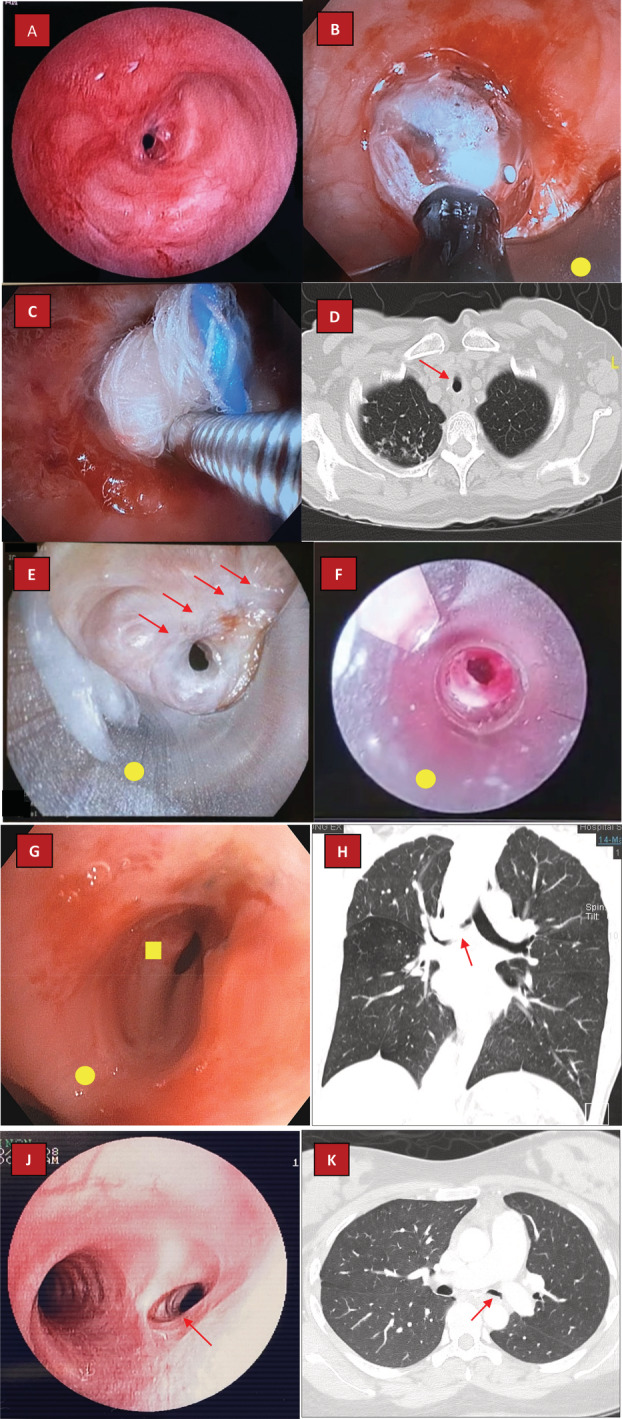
Computed tomography (CT) and bronchoscopy images. (A) Flexible bronchoscopy demonstrating short‐segment concentric membranous tracheal stenosis with opening of approximately 2 mm. (B) Attempting balloon dilatation of stenotic segment under rigid bronchoscopy (bevel of rigid bronchoscope marked with yellow circle). (C) Application of topical mitomycin‐C (TMC) at stenotic segment using soaked gauze balls post balloon dilatation. (D) CT demonstrating stenosis at mid‐trachea (red arrow) due to endobronchial tuberculosis (EBTB). (E) Bronchoscopy demonstrating severe tracheal stenosis with opening of 2 mm. A dense, pale, and thick fibrotic band (red arrows) was seen immediately anterior to the luminal opening. Bevel of rigid bronchoscopy marked with yellow circle. (F) Attempting mechanical coring and dilatation of tracheal stenosis by using a rigid bronchial tube (yellow circle). (G) Bronchoscopy showing bronchomalacia and stenosis of the right main bronchus (yellow square); main carina marked with yellow circle. (H) Right main bronchus stenosis (red arrow) as demonstrated on CT. (J) Surveillance bronchoscopy 15 months post balloon dilatation and TMC showing a narrowed but patent right main bronchus (red arrow). Right main bronchus was severely stenosed with a luminal opening of 2 mm pre procedure. The patient remained well. (K) CT showing a narrowed left main bronchus due to EBTB (red arrow).
